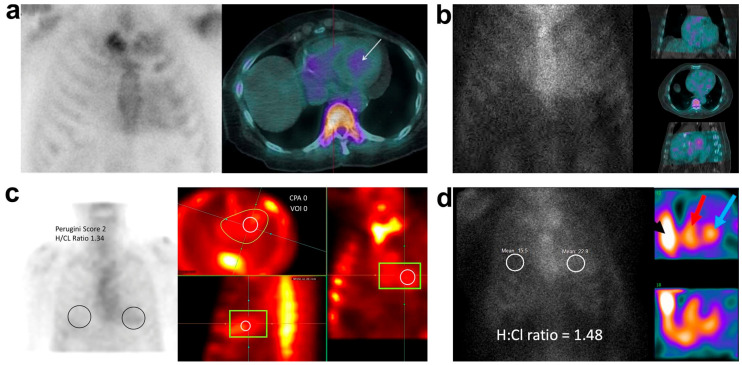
“False positive” interpretations on ^99m^Tc-PYP planar images. (a) A planar scan was performed at 1 h and interpreted as Grade 2 uptake using a visual grading scale relative to ribs (VGSr), with a heart-to-contralateral (H/CL) ratio of 1.5. However, ^99m^Tc-PYP scan at 3 h showed Grade 1 planar uptake (VGSr), with radiotracer within the left ventricular blood pool (white arrow) but no myocardial uptake on SPECT/CT images [7]. (b) A “positive” planar study at both 1 and 3 h was determined based upon Grade 2–3 uptake (VGSr) and H/CL ratio of 1.63 and 1.48, respectively. However, this “false positive” interpretation was refuted by Grade 1 uptake referring to visual grading scale relative to upper segment of sternum (VGSs) approach, with concordant to no radiotracer myocardial uptake on SPECT/CT images [11]. (c) In an AL-CA patient, planar imaging at 3 h was interpreted as “positive”, based upon Grade 2 uptake (VGSr) with a H/CL ratio of 1.34 (black circle). No radiotracer myocardial uptake (the range between green box/irregular circle and white circle) was found on SPECT images, and this “false positive” interpretation can be also refuted by using the VGSs approach [14]. (d) A “positive” planar study at 3 h was determined based upon Grade 2 uptake (VGSr) and H/CL ratio of 1.48 (white circle). Only blood pool activities of right (red arrow) and left (blue arrow) ventricles, and intense radiotracer uptake in the sternum (black arrow) were indicated on 3-h SPECT images [31]. Grade 1 uptake using the VGSs approach could reclassify this case as “false positive” as well. (All the reprint permissions have been obtained from the publishers).
